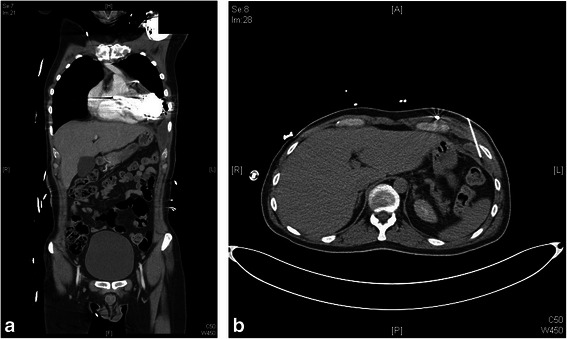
a CT showing abscess reaching up to and adjoining the HVAD. b CT showing CT-guided abscess drainage
